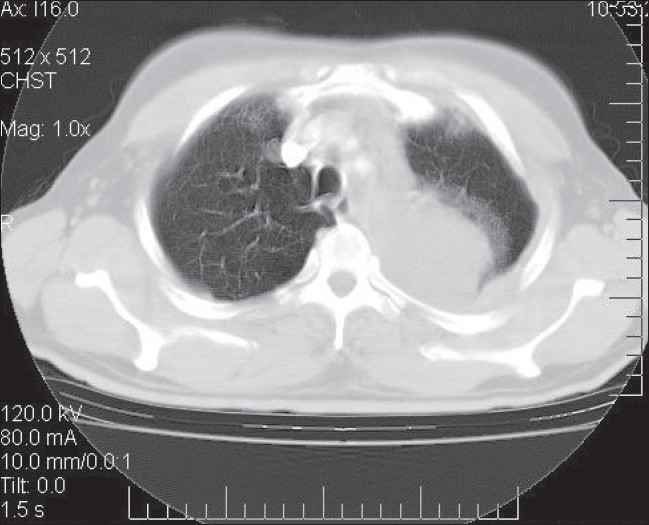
CT scan showing opaque left hemithorax with pleural effusion
